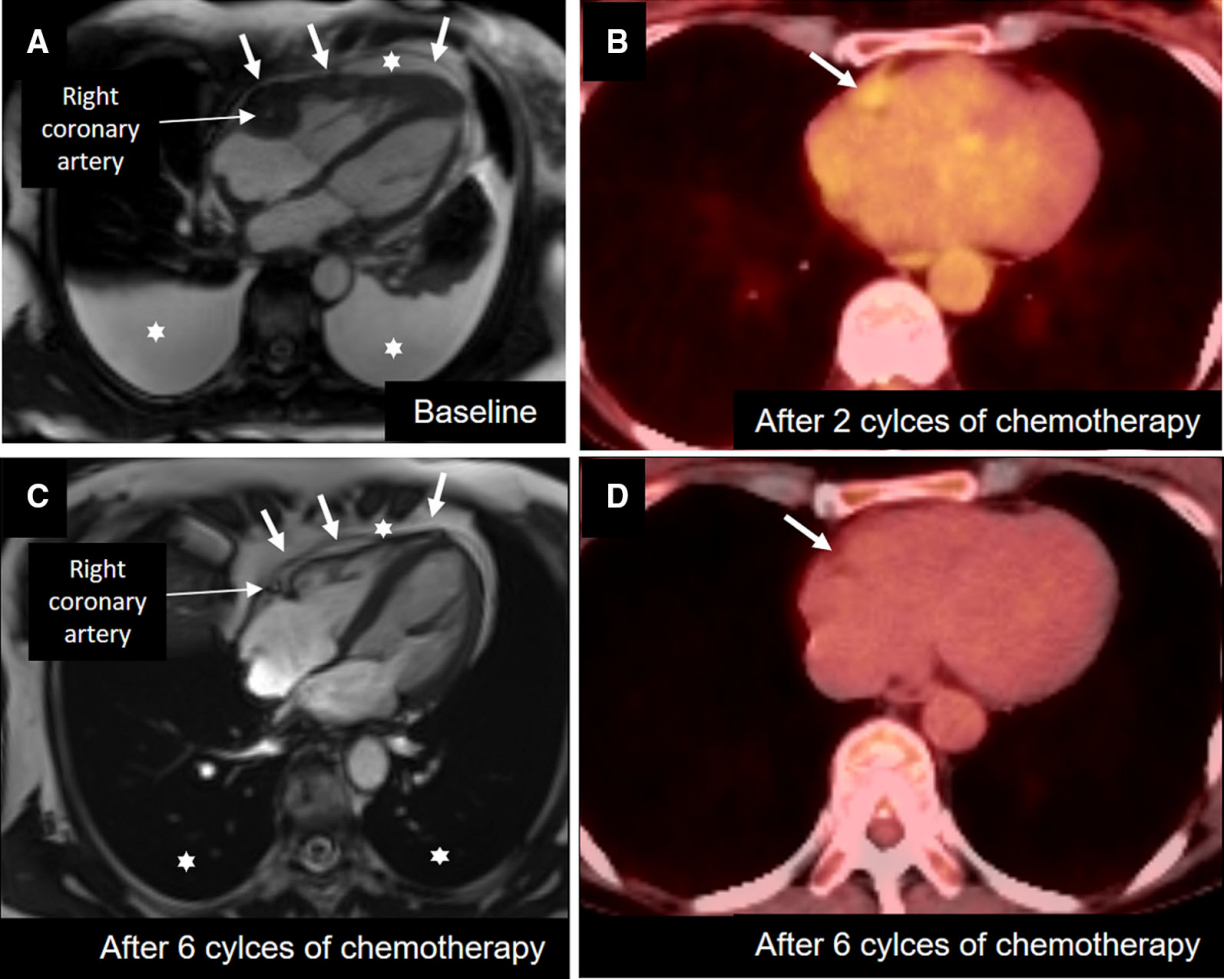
Baseline and follow-up CMR and 18F-FDG PET/CT imaging. A Steady-state free precession-cine imaging shows a defined, heterogeneous myocardial mass (arrows) involving the right atrial ventricular groove, surrounding the right coronary artery and extending to the right ventricle wall, as well as moderate pericardial and pleural effusion (asterisks). CMR is the ideal modality to differentiate angiosarcoma (i.e., typically involving the right atrial appendage and not surrounding the right coronary artery, central liquefaction necrosis with rim enhancement with lack of central enhancement) from lymphoma like in our case with typically diffusely involvement of the right atrioventricular groove and completely surrounding the right coronary artery, extending towards the right ventricle and mostly homogenous perfusion and heterogenous late gadolinium enhancement. B PET/CT shows little ^18^F-FDG uptake after 2 cycles. C and D CMR and ^18^F-FDG showed complete remission after 6 cycles of chemotherapy
